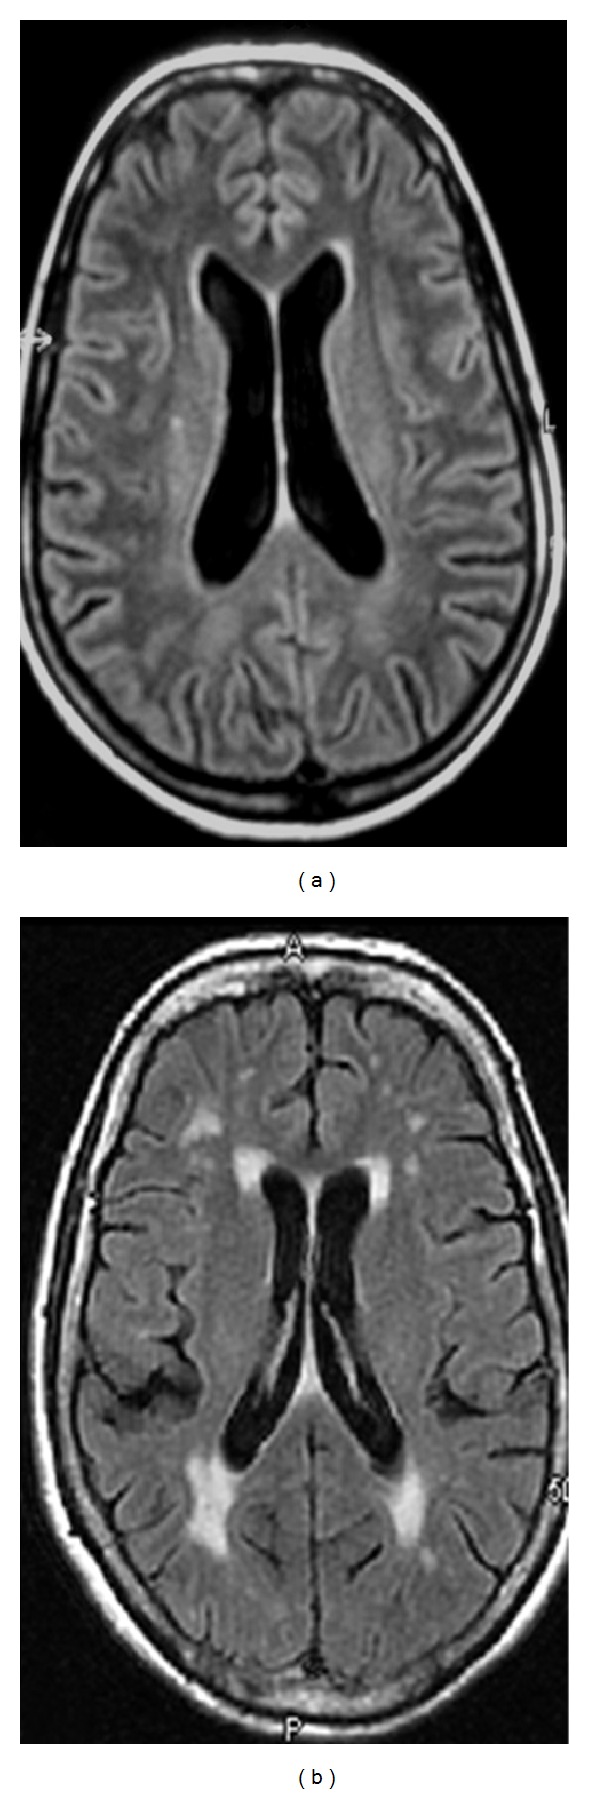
Axial brain FLAIR-magnetic resonance imaging of two female patients with primary Sjögren's syndrome. Patient (a) had three cognitive subtests below the 5th percentile and a Wahlund score of 1 for white matter lesions. Patient (b) had five cognitive subtests below the 5th percentile and a Wahlund score of 2 (beginning confluence of lesions) for white matter lesions.
